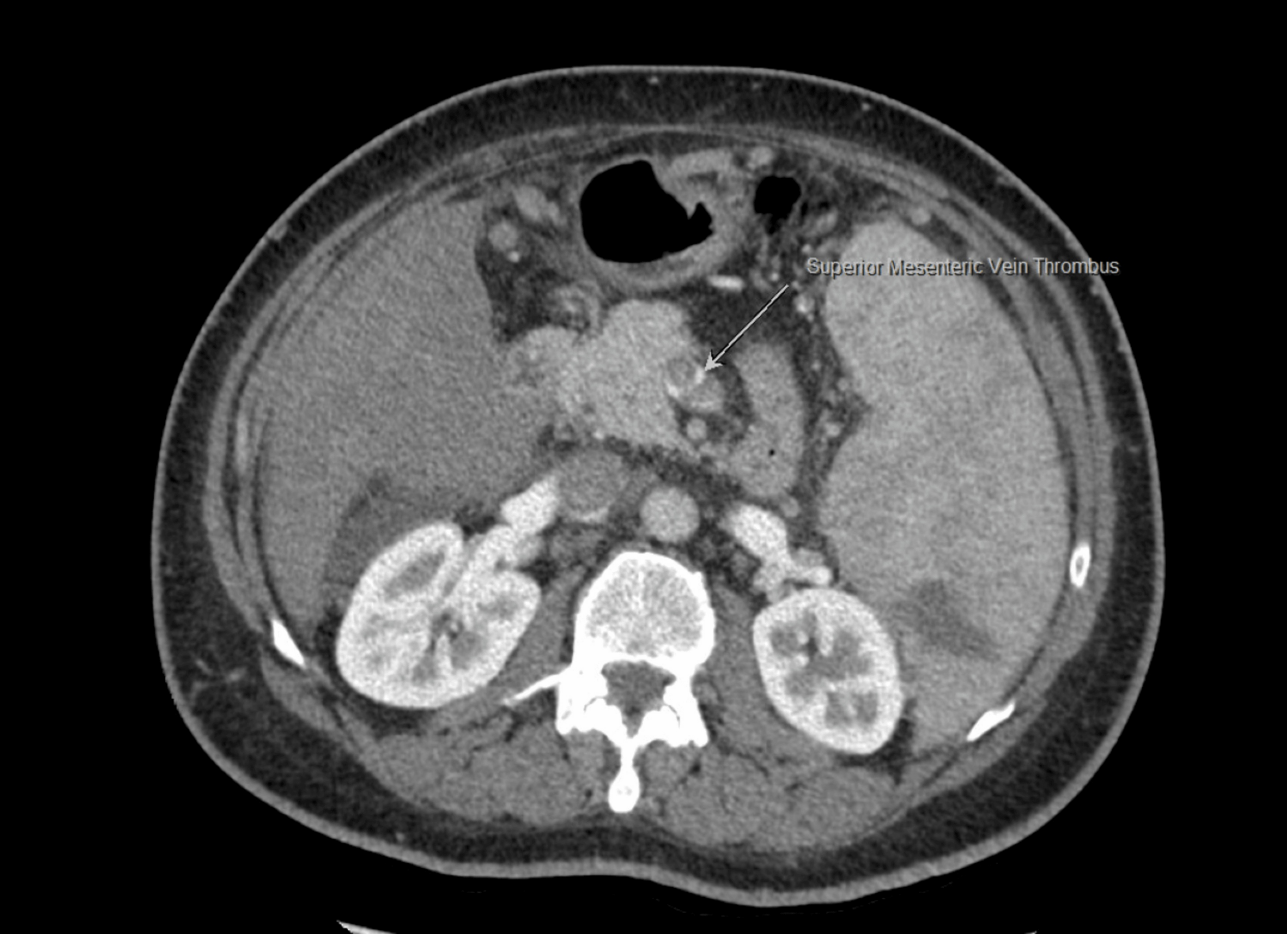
CT abdomen revealed a thrombus in the superior mesenteric vein (marked by the white arrow)
